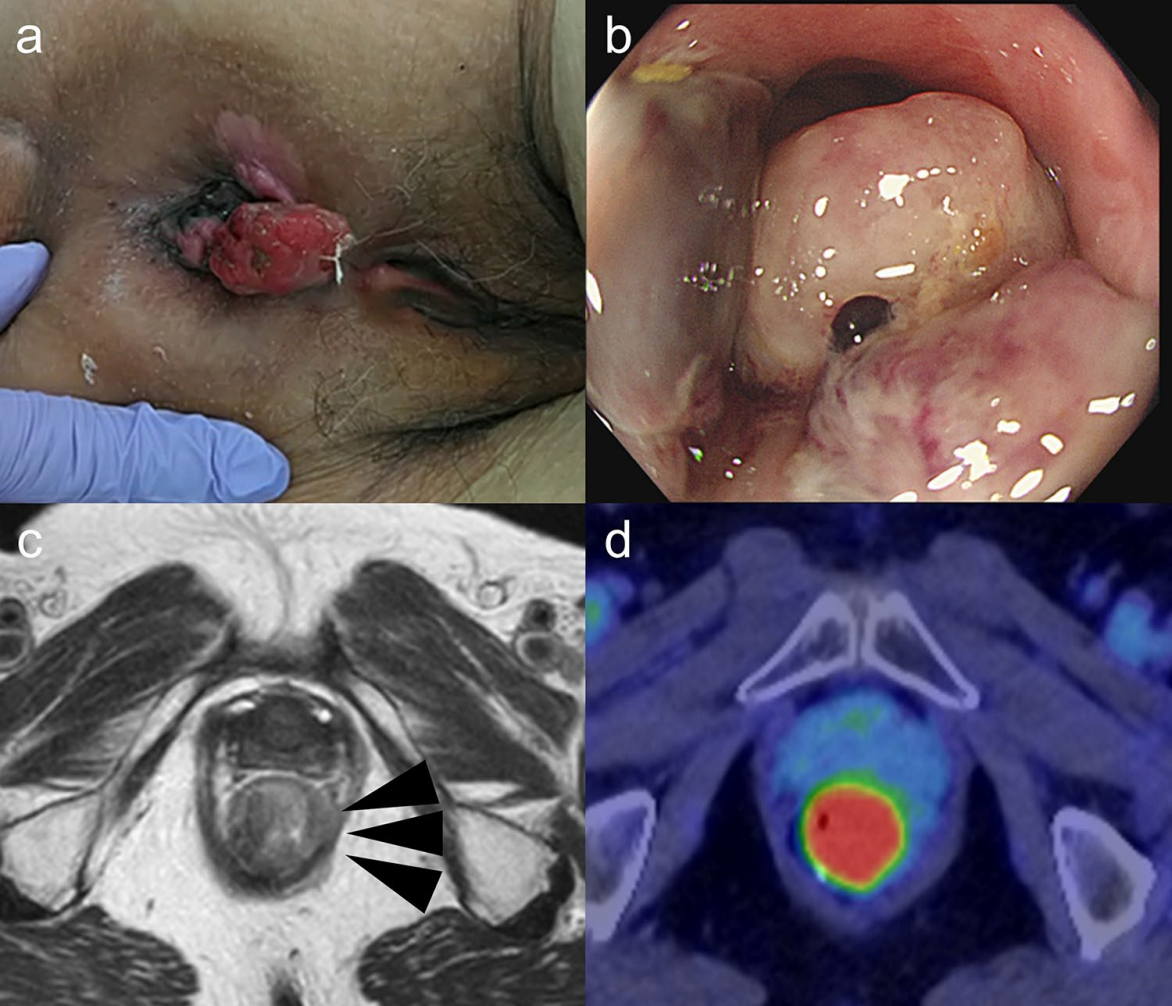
Results of the examination before the second surgical treatment. a Picture of the anorectal lesion. Anorectal lesion protruding from the anus. b Colonoscopy, black elevated lesion at the anastomotic site over the anal verge. c MRI findings, axial T2-weighted imaging showing a hyperintense signal. d PET, anastomotic lesion with an abnormal accumulation of Max SUV: 15.3
